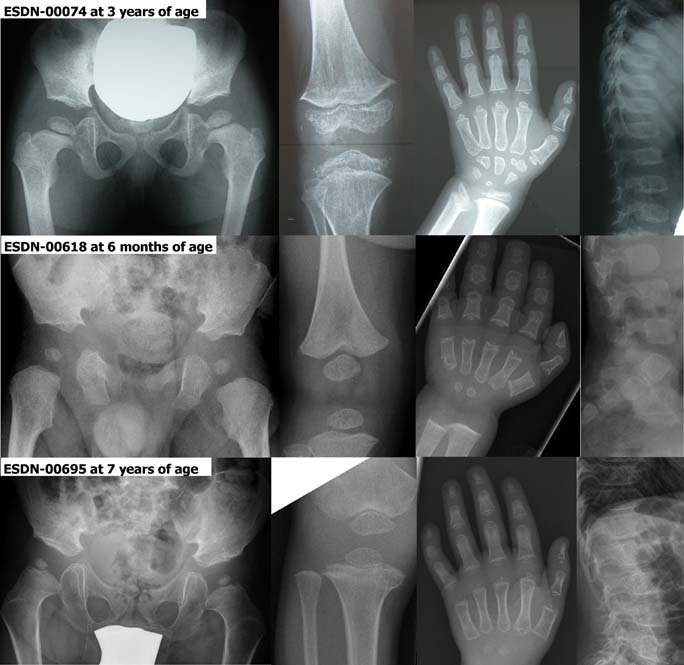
Radiographic findings in *COMP* negative patients referred as PSACH. ESDN-00074: Radiographs of the spine, pelvis, knees, and left hand. With the exception of the left hand taken at 4 years of age, all radiographs were taken at the age of 3 years. The vertebral bodies are flattened, the proximal femoral epiphyses are small, and the femoral necks are short. The trochanters minor are well ossified and prominent present. The knee epiphyses are small and irregularly ossified. The metaphyses in the knees are widened and the femoral distal metaphyses have spikes at both ends. The submetaphyseal regions have a striated pattern. The hands show shortening and broadening of the metacarpals and phalanges with small epiphyses. The epiphyses of the proximal phalanges are fragmented. There is advanced carpal ossification with rather rectangular (and not rounded) shape of the carpal bones. The distal ulna shows precocious ossification of the epiphysis and cupped metaphysis. ESDN-00618: Radiographs of spine, pelvis, knee, and hand taken at the age of 6 months. The pelvis is abnormal with flat and trident acetabular roof and small and broad iliac wings. The ischiadic bones are broad. The proximal femoral epiphyses are well ossified for age. The femoral necks appear broad. The hand shows shortening of phalanges and metacarpals, especially the proximal and middle phalanges are very short with poor diaphyseal modeling and precocious ossification of the epiphyses that are attached to the metaphysis. The vertebral bodies are mildly foreshortened with posterior scalloping. No gross abnormalities are seen at the knee. ESDN-00695: Radiographs of spine, pelvis, knee, and hand taken at the age of 7 years. The mini-epiphyses in the hips and the small epiphyses in the knees with translucent submetaphyseal areas in the proximal tibia are reminiscent of PSACH. However, the hand shows only mild shortening of the phalanges and metacarpals. In addition, there is marked delay in carpal ossification. The epiphyses in the wrist and hands are too small for age. The vertebral bodies are flattened and elongated.
